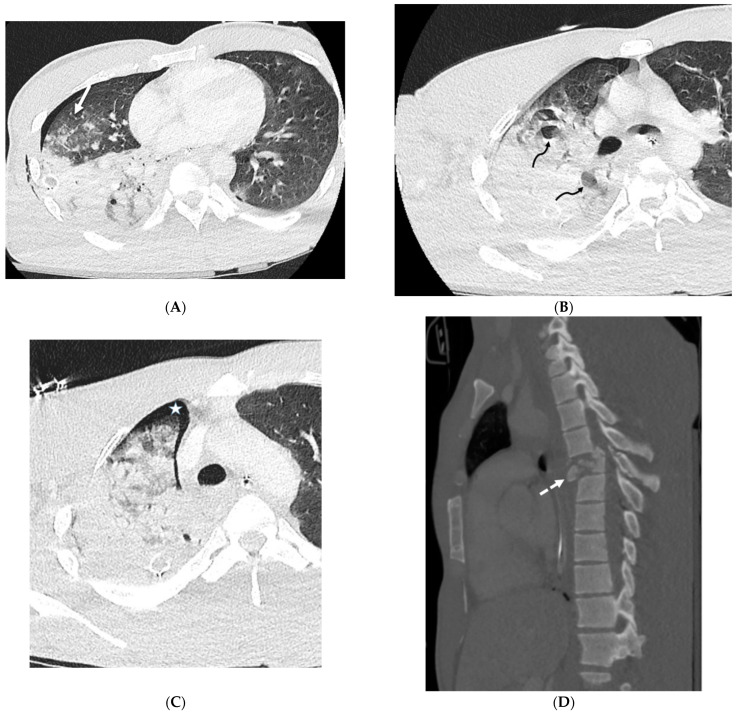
A 28-year-old male admitted after a motorcycle crash. Axial (A–C) and sagittal (D) chest CT images demonstrate interlobular septal thickening (solid white arrow) and airspace opacities in the right lung compatible with severe pulmonary hemorrhage and contusions. Pulmonary lacerations are seen as focal lucencies (curved black arrows) in the right upper and right lower lobes. Note small right pneumothorax (white star) and right chest wall subcutaneous emphysema as well. A sagittal chest CT image (D) shows an acute comminuted fracture of the T6 vertebra with retropulsion and spinal malalignment (dashed white arrow).
